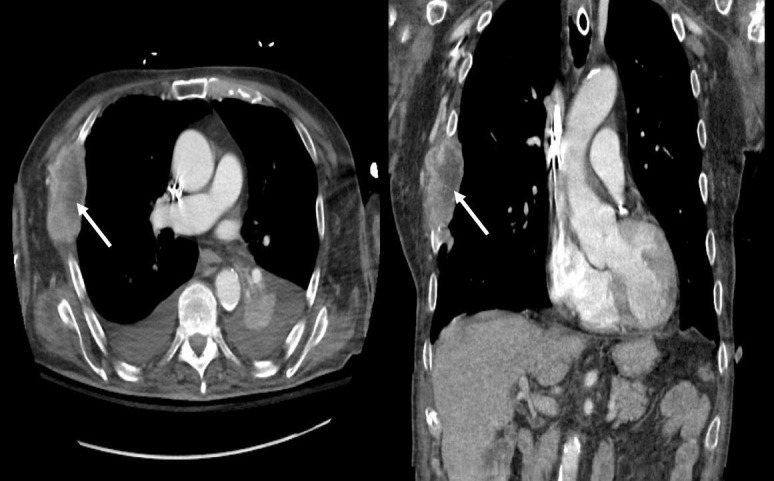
Computed Tomography of the Chest with Lytic Lesion on Right Ribs
